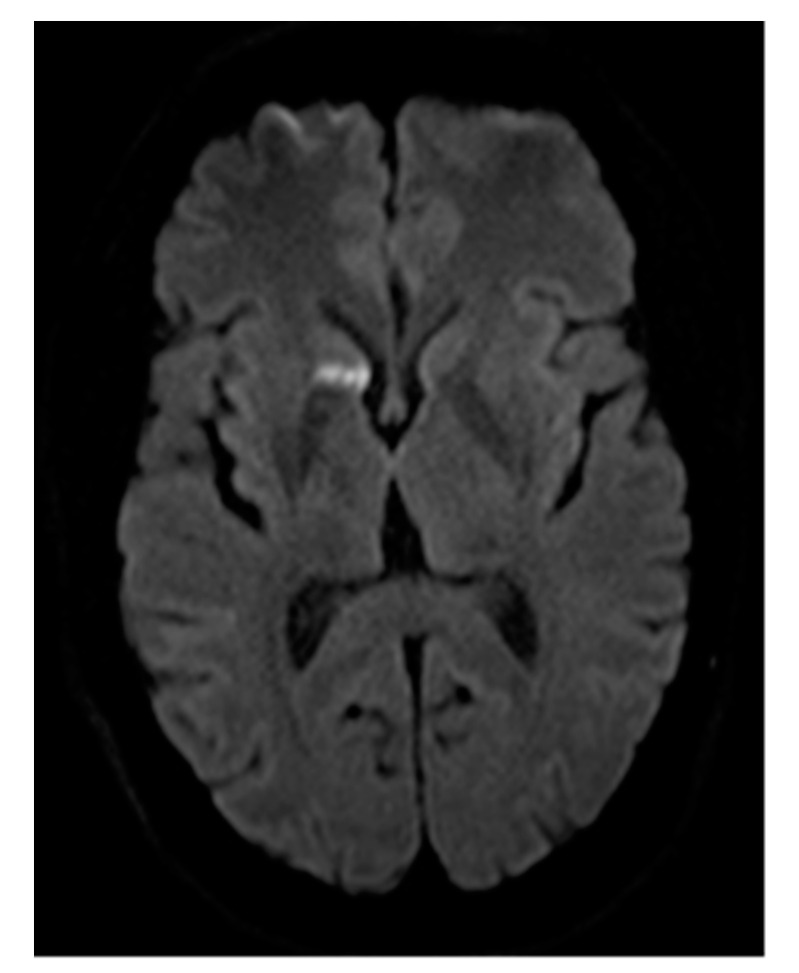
Cerebral DWI (b = 800) demonstrating subacute cerebral ischemia, in this case prior to embolization of multiple PAVMs. The newly developed lesion arose between screening for PAVM and the day of interventional therapy, but the patient did not exhibit any clinical signs or symptoms. This example highlights the importance of DWI for the detection of pre-existing cerebral ischemia, as well as peri-interventional cerebral insult.
